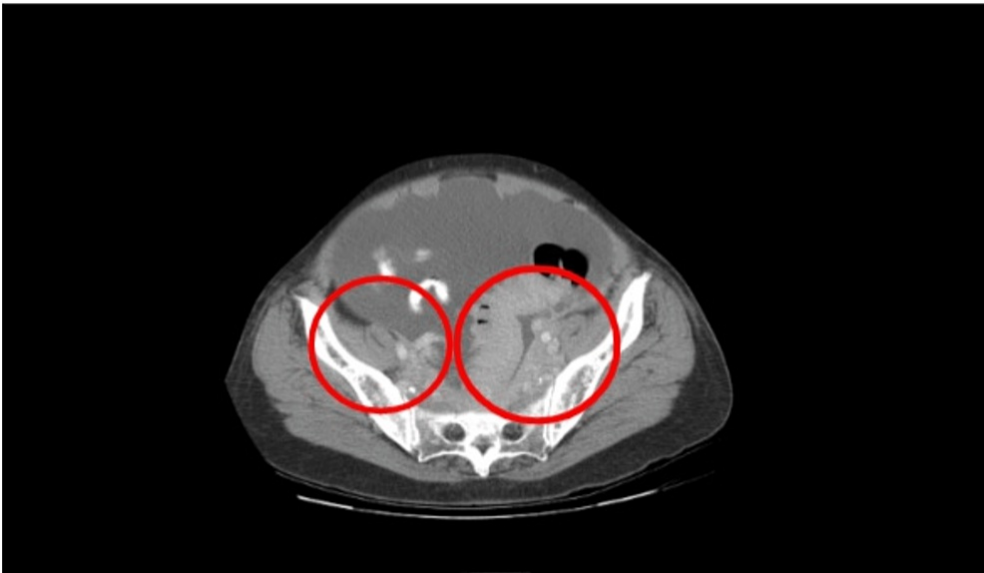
CT abdomen/pelvis with oral and IV contrast Extensive soft tissue masses encasing the mesenteric vessels, omentum, retroperitoneal, and sigmoid colon and rectum (red circles)
